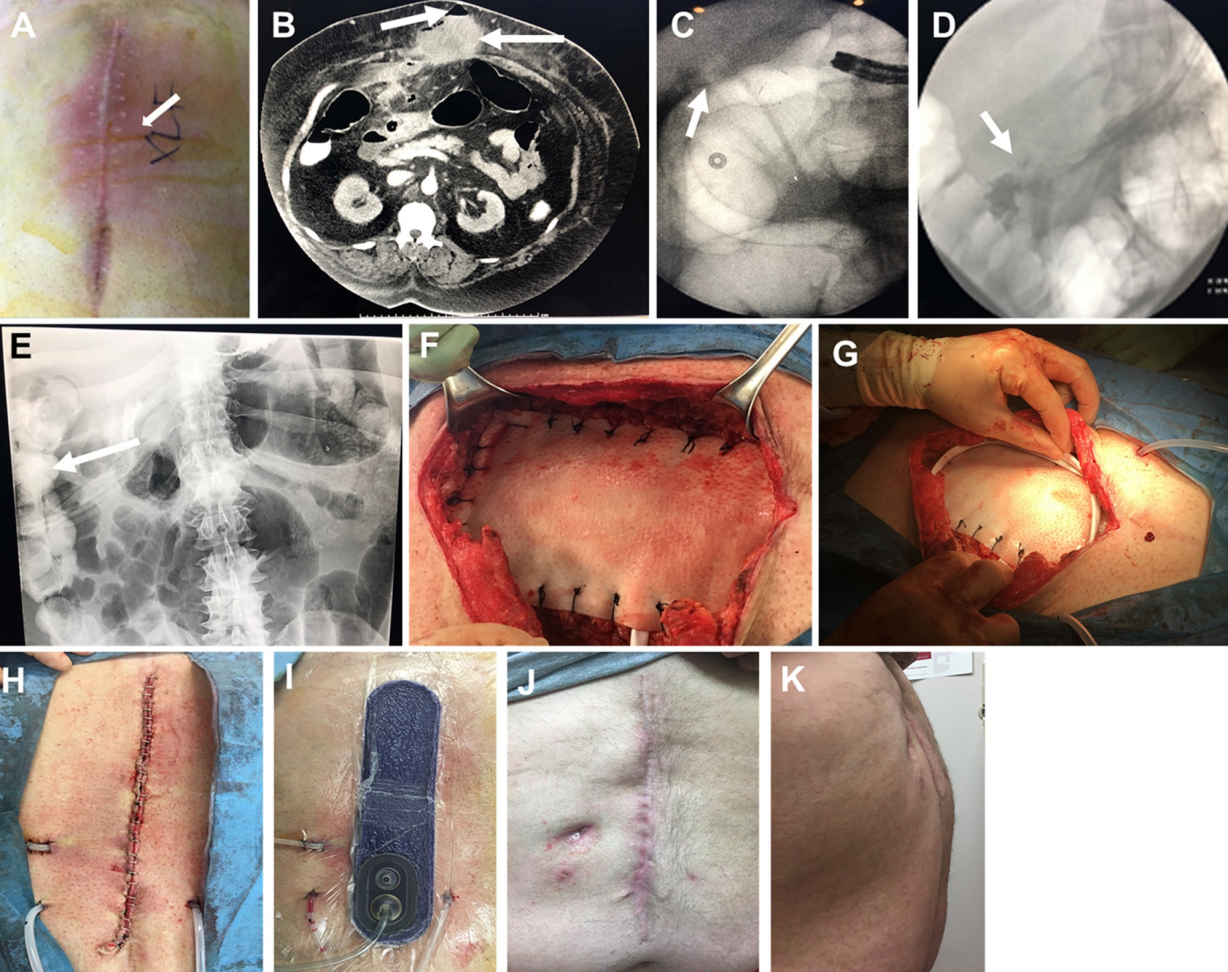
Open abdomen repair and instillation of a contaminated, frozen abdomen A. Midline erythema; B. Abdominal CT showing potential bowel perforation (left arrow) and abdominal abscess (right arrow); C. Endoscopic duodenal stent placement; D. Elimination of duodenal leak achieved (arrow); E. Duodenal stent in right colon (arrow); F. Application of reconstruction tissue matrix; G. Drain application; H. Closed abdominal incision, I. Application of closed incision negative pressure therapy; J. Anterior view of fully healed abdominal incision; K. Lateral view of fully healed abdominal incision
